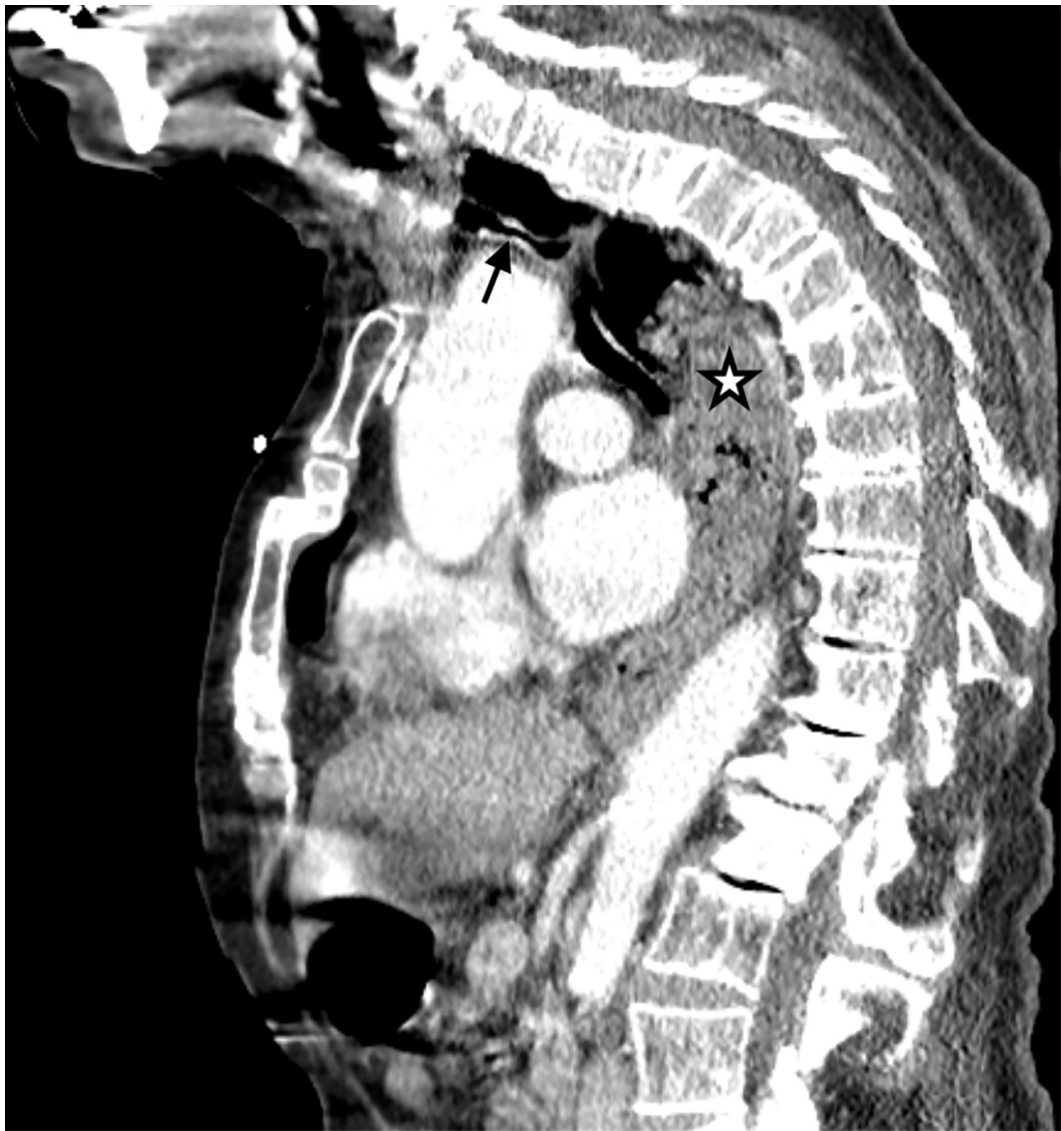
Computed tomography (sagittal view) demonstrating luminal narrowing of the trachea (black arrow) with marked distention of the esophagus. Significant inspissated material noted throughout the distended esophagus (white star).
